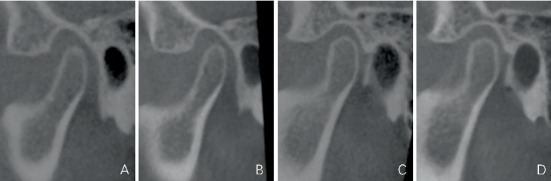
Sagittal view of TMJ on CBCT. (A) Pretreatment right TMJ. (B) Posttreatment right TMJ. (C) Pretreatment left TMJ. (D) Posttreatment left TMJ.
